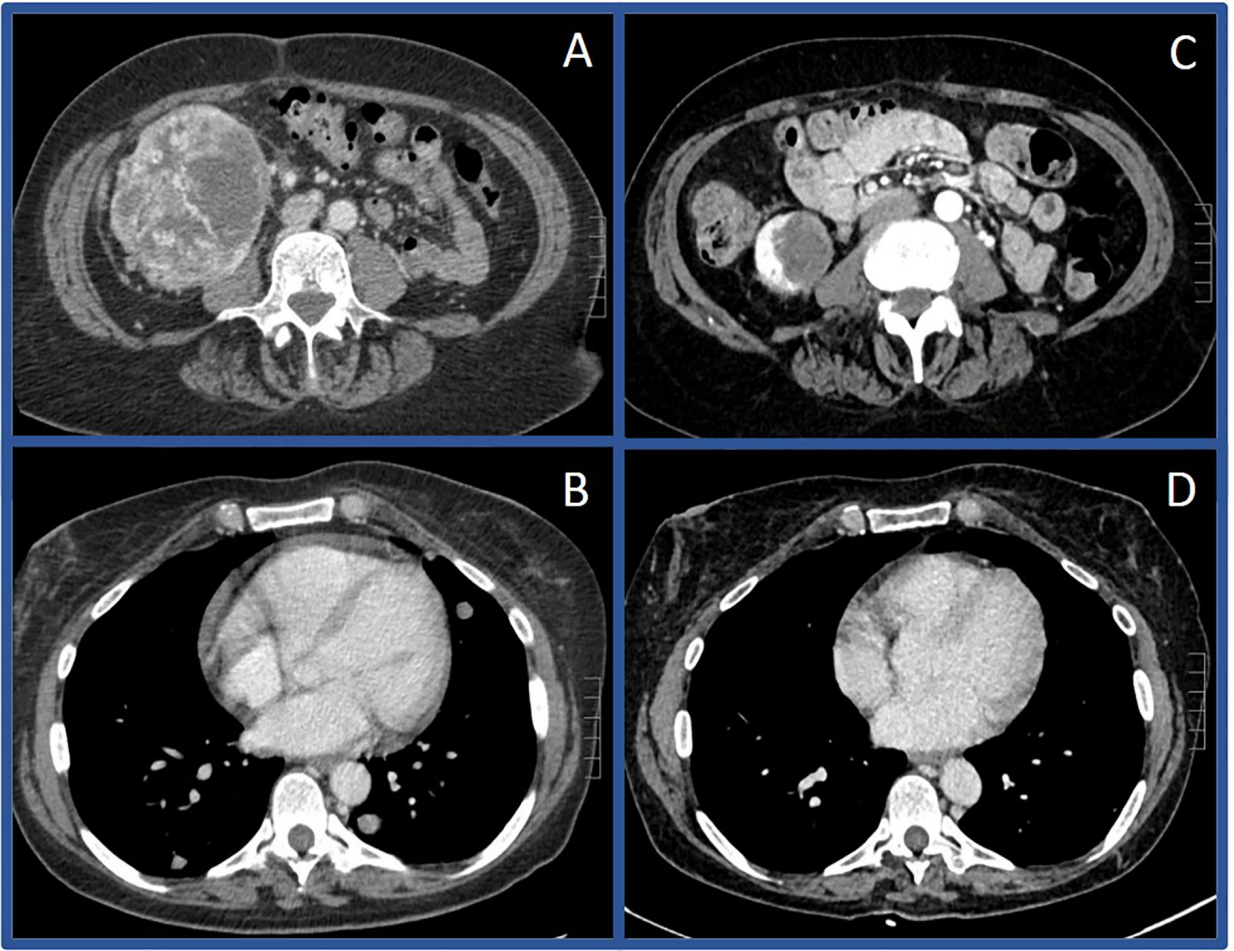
Computerized tomography scans before and after immunotherapy in Case #2 Initial CT scan in July of 2018 showing large right renal mass (A) and pulmonary metastases (B) compared to CT scan in December of 2019 showing substantially decreased mass (C) and resolution of pulmonary metastases (D).
